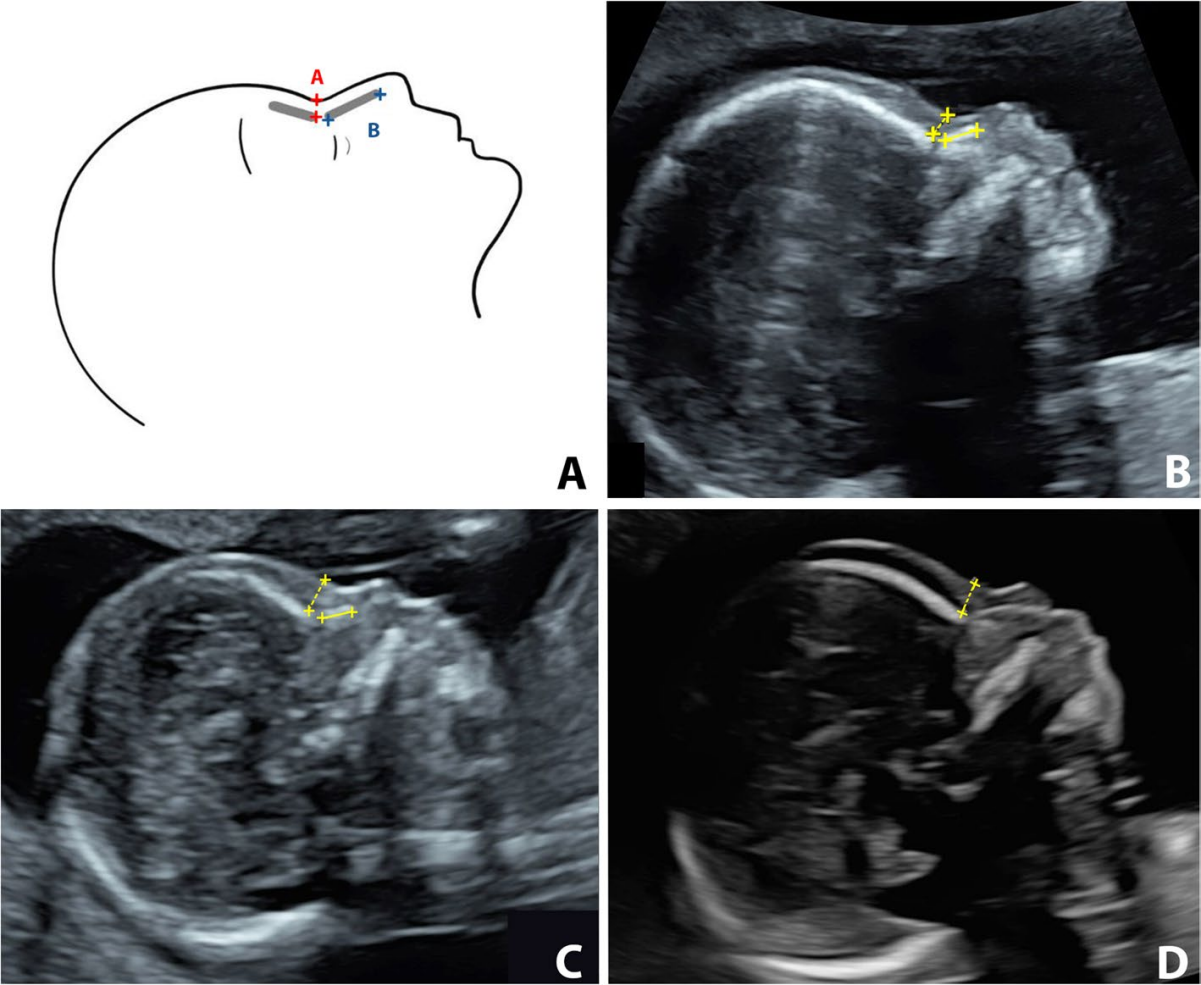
Measurement of mid-facial plane facial ultrasound markers. Legend: The landmarks used for measurement of the facial ultrasound markers in the study are shown in (A). PT is the distance between the edge of the lowest part of the frontal bone and the facial skin anteriorly (A), NBL is measured at the ossification line along the fetal nose (B). Also shown are ultrasound images of the mid-facial plane in a euploid fetus (B) and a Down syndrome fetus with a hypoplastic nasal bone (C) and absent nasal bone (D). PT: prenasal thickness; NBL: nasal bone length.
